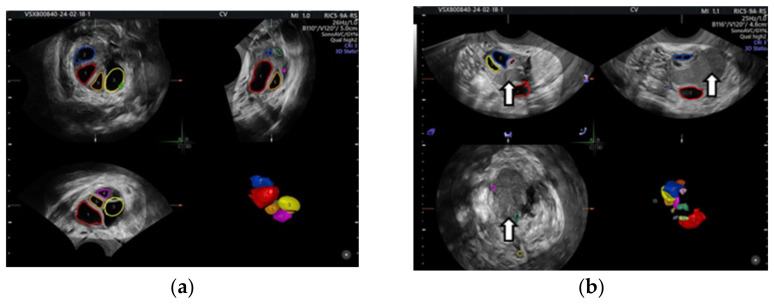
Color-coded stimulated follicles in the IVF cycle: (a) right ovary with normally growing follicles, (b) in the left ovary the arrows show an ovarian endometrioma compressing the follicles—vaginal 3D ultrasound with SonoAVC software (SonoAVC, automatic volume calculation: GE Medical Systems).
